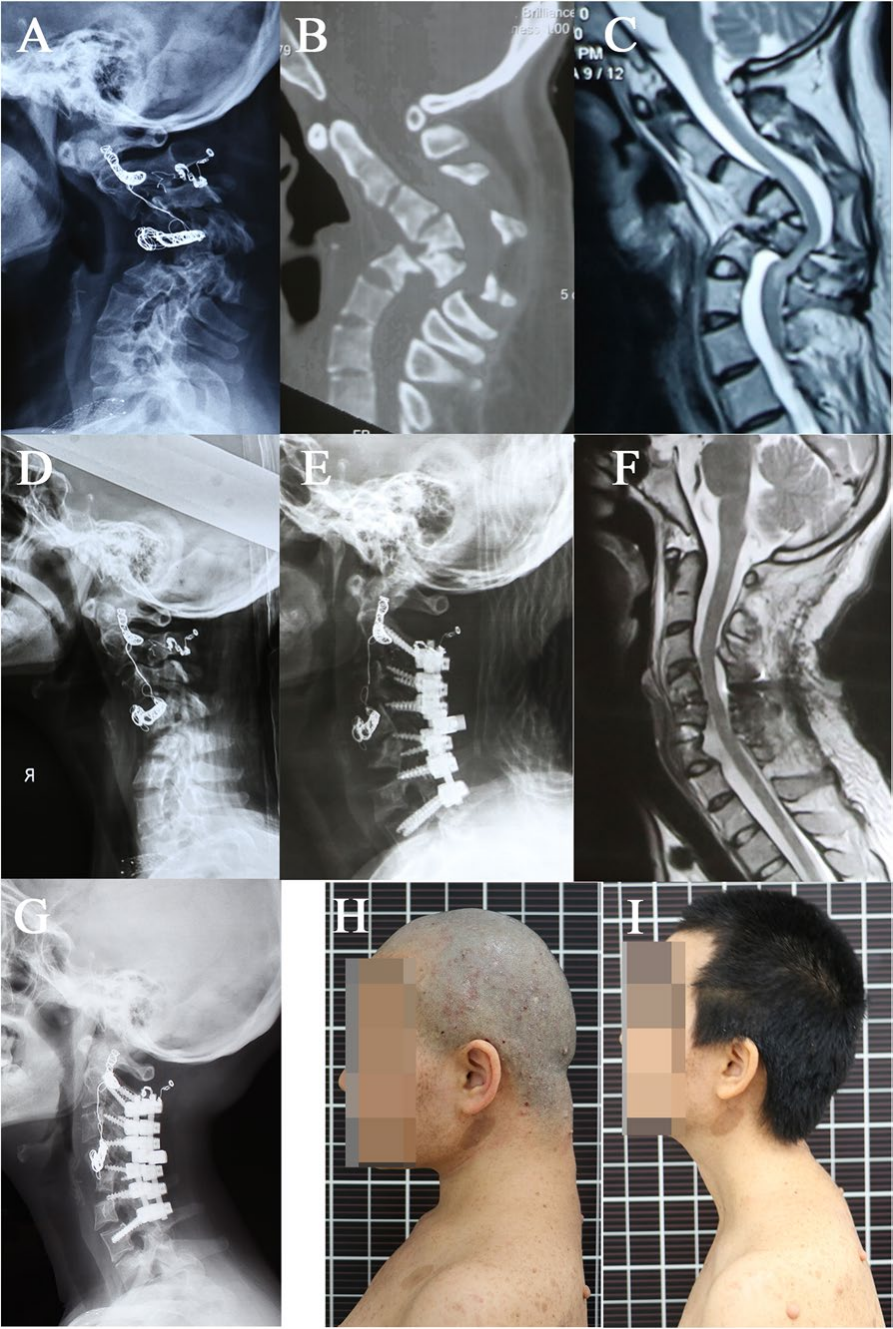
A 29-year-old case with NF-1 cervical kyphosis. Preoperative JOA score was 12. A-C Preoperative X-rays, CT showed severe kyphosis of 86° with severe dystrophic changes in cervical spine, and MRI demonstrated a mild compression from kyphosis. D After halo-traction, the kyphotic angle gradually improved from 86° to 45°. E-F Cercical kyphosis improved to 8° after surgery, and MRI showed the compression of spinal cord which was decompressed. G X-ray showed a solid bone union and no significant correction loss at follow-up. H-I Pre-op and latest follow-up clinical photos
